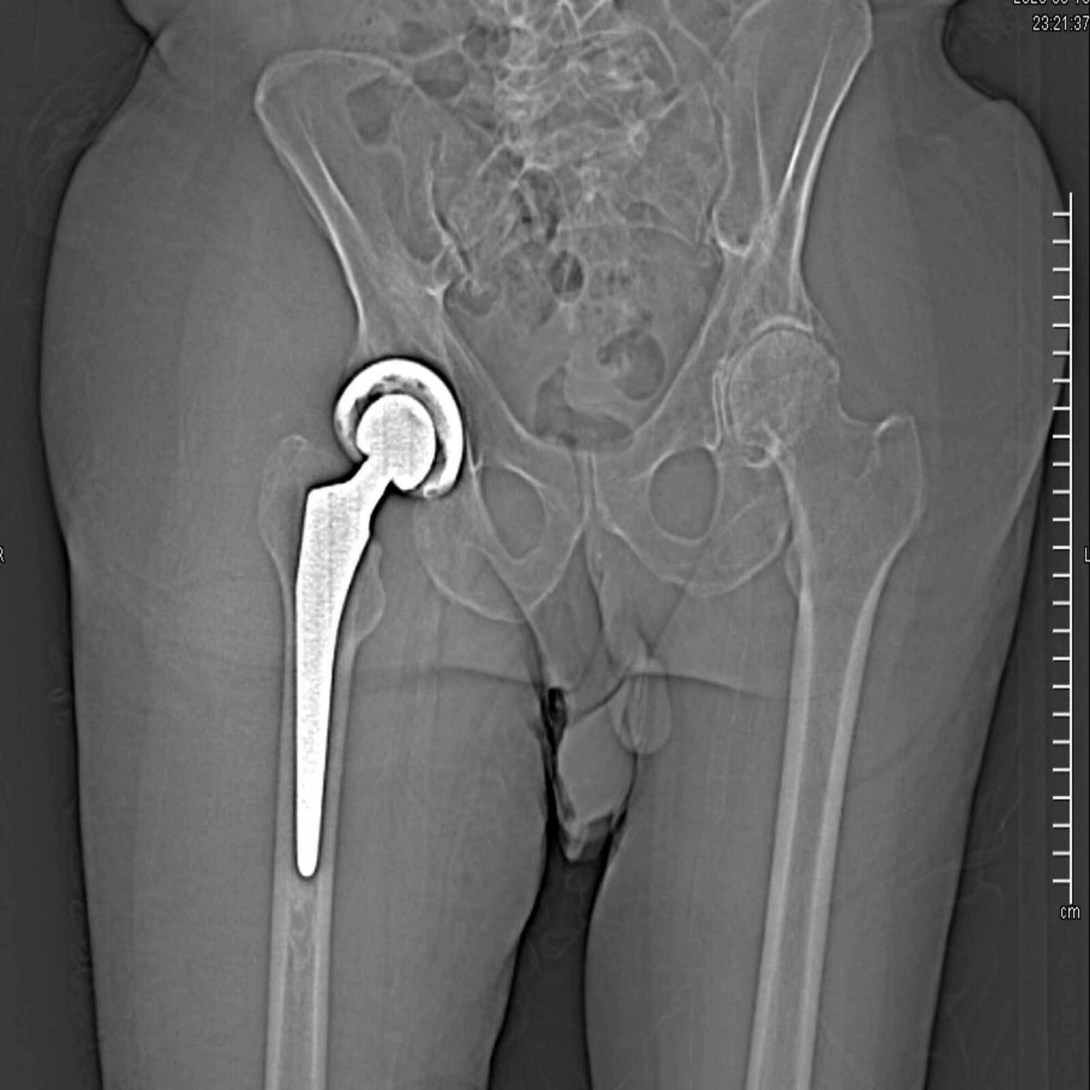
CT showing right hip hematoma extending to the thigh
